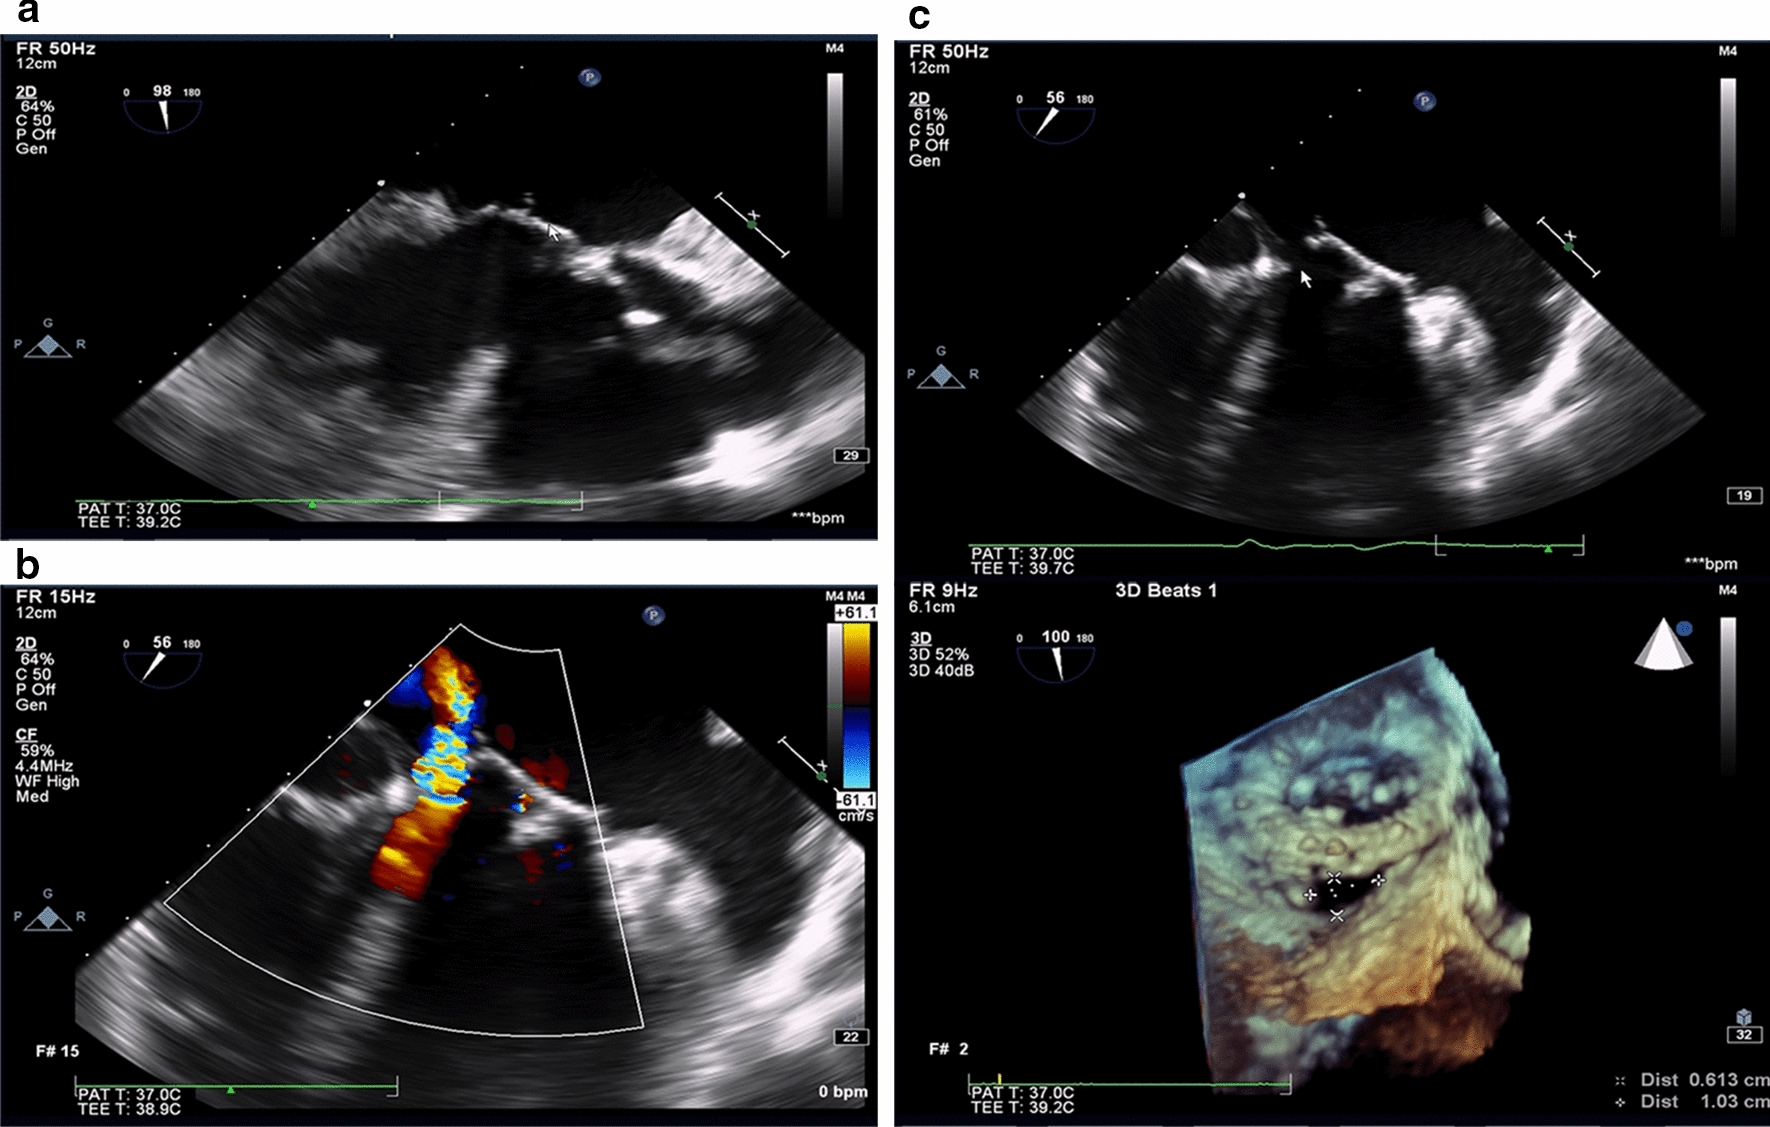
The *Candida parapsilosis* complex prosthetic valve endocarditis of case 2. a Echocardiography showed a short abnormal strip about 5mm×3mm on the prosthetic mitral valve of the left atrium side; b The Color Doppler showed perivalvular leakage (moderate-severe); c Transesophageal 3D image showing a bioprosthetic mitral valve. A perivalvular crack about 10.3×6.1 mm could be seen around the medial side of the prosthetic mitral valve
